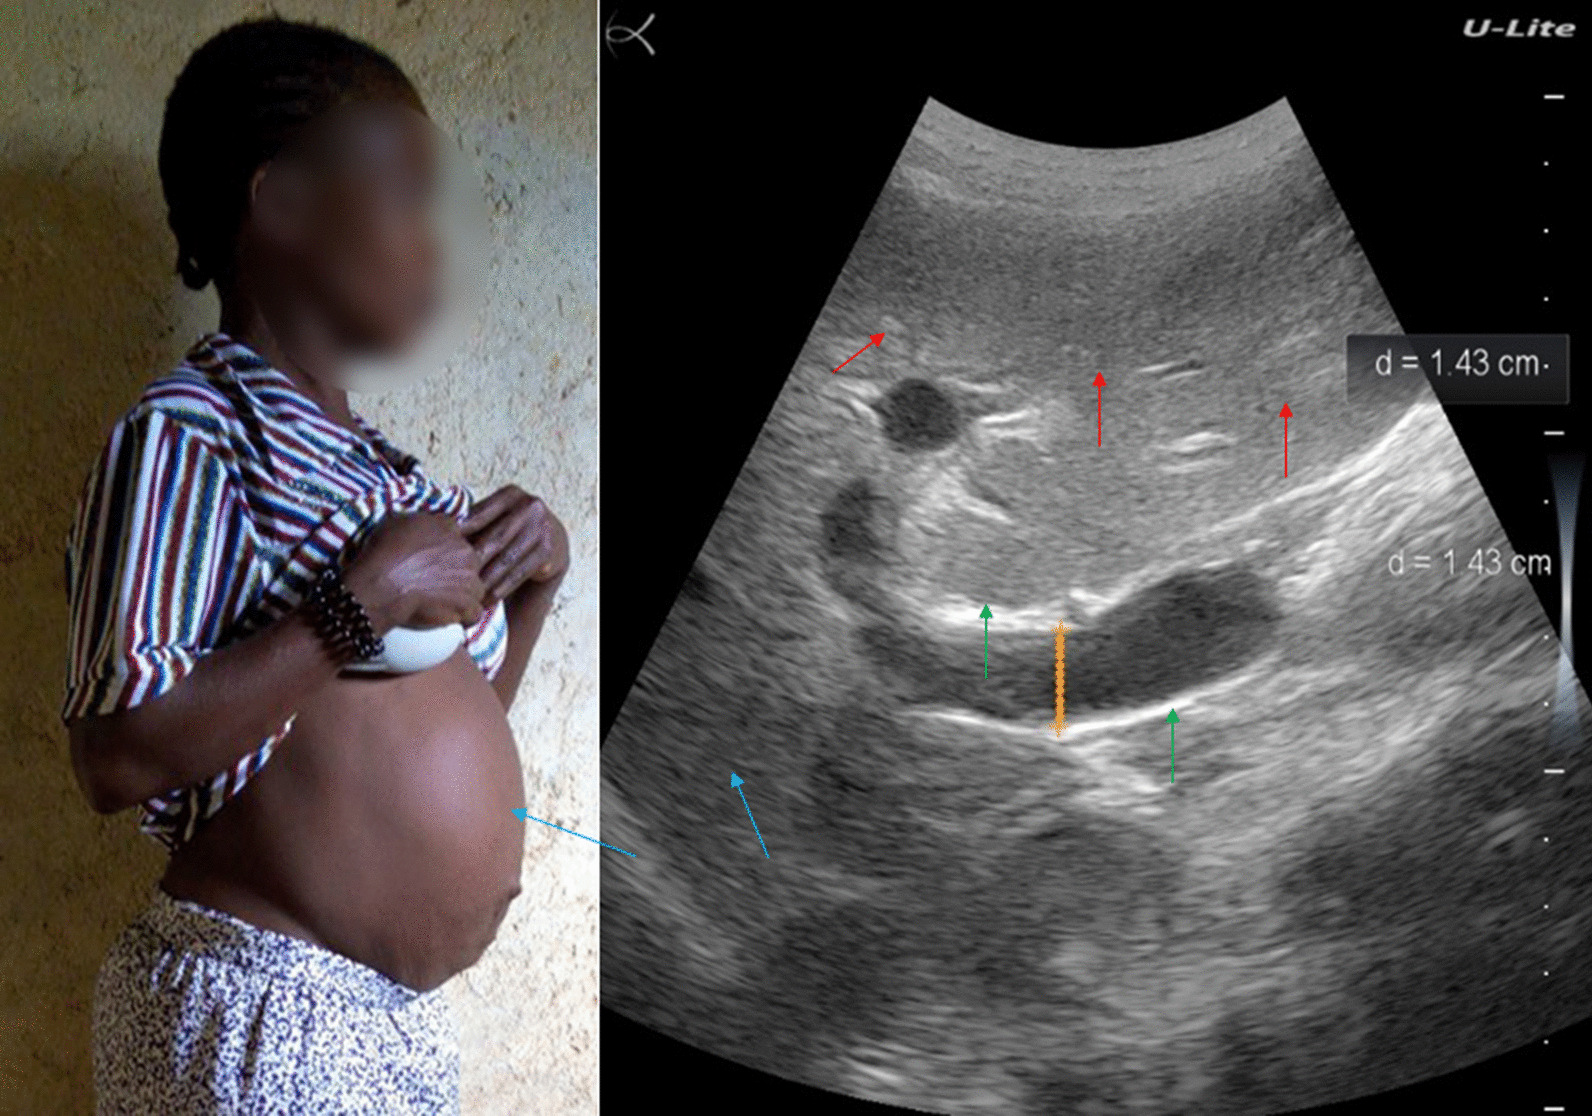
Patient #7, woman with a big belly (blue arrow, left). Ultrasound examination (right) showing liver with distorted architecture, nodular surface, heterogenous echotexture of the parenchyma (red arrows), decreased definition of the portal vein wall (green arrow), and ascites (blue arrow)
